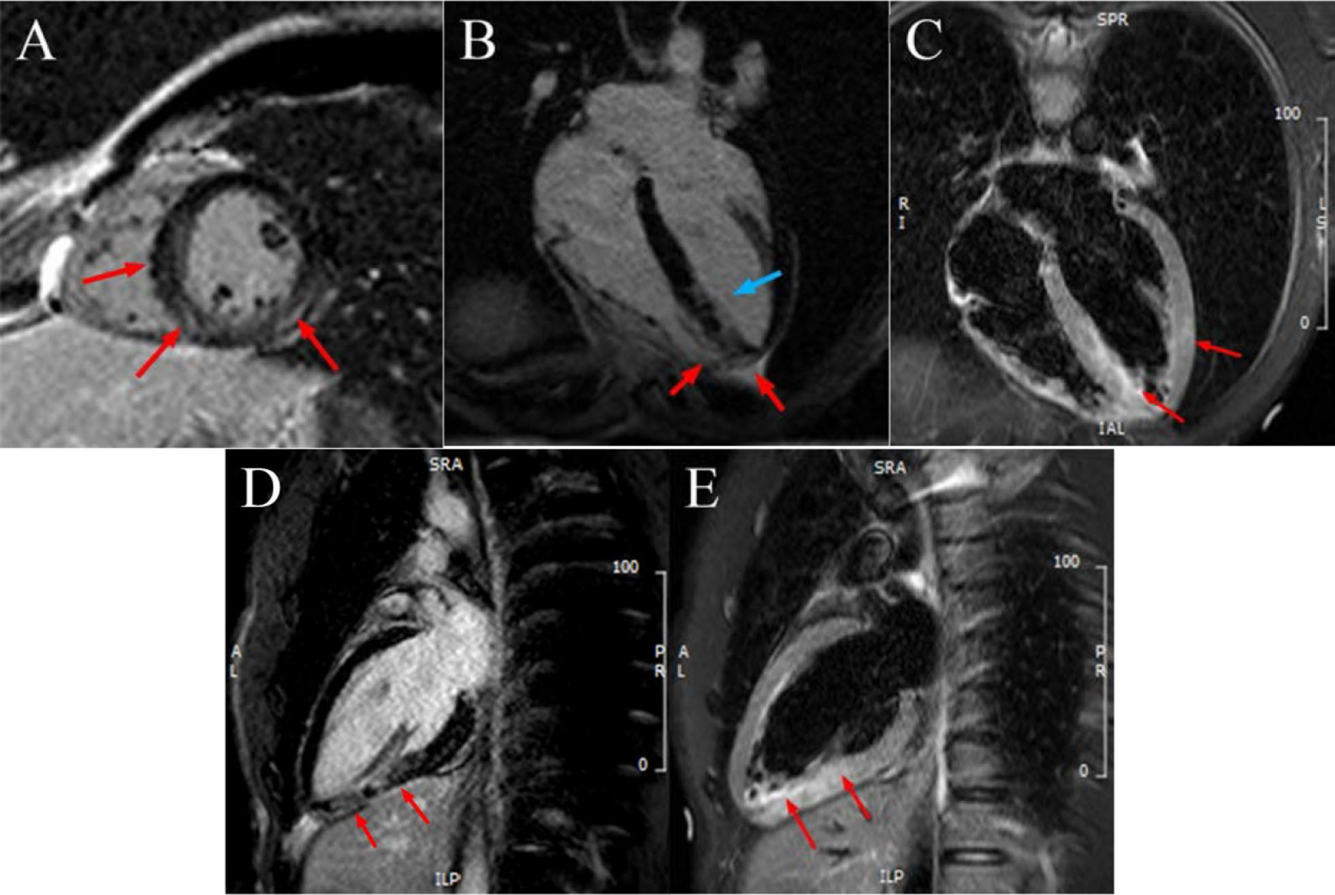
Cardiac MRI of patient. A CMR late gadolinium in SAX view showed patchy LGE of the mid to apical inferior and septal segments; and pericardial enhancement in the inferior and inferolateral wall, B LGE of the mid to apical inferior and septal segments at 4 chambers, C Septal and lateral hyper-enhancement at T2W STIR 4 chambers, D LGE of the mid and apical inferior at 2 chambers, E Mid and apical inferior hyper-enhancement T2W STIR 2 chambers
